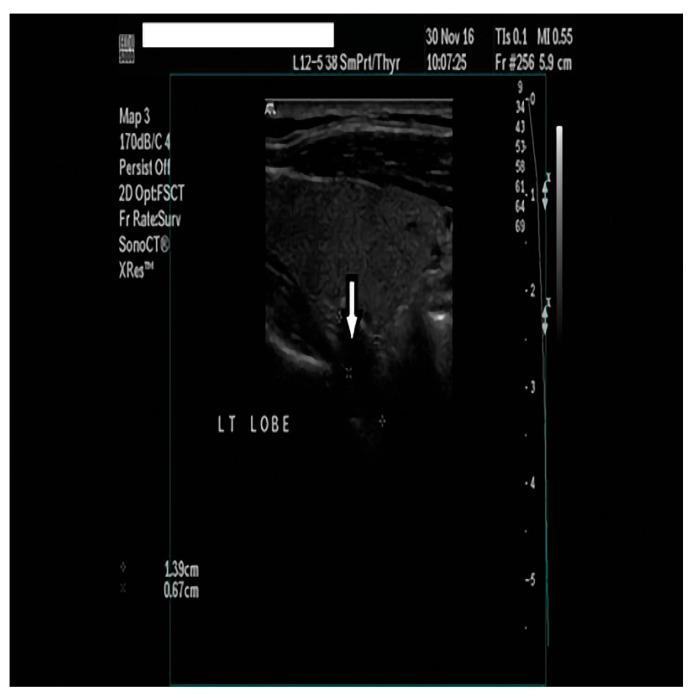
A neck ultrasound revealed a 1.4 × 0.7 × 0.5 cm oval-shaped, hypoechoic mass below the left thyroid lobe.
